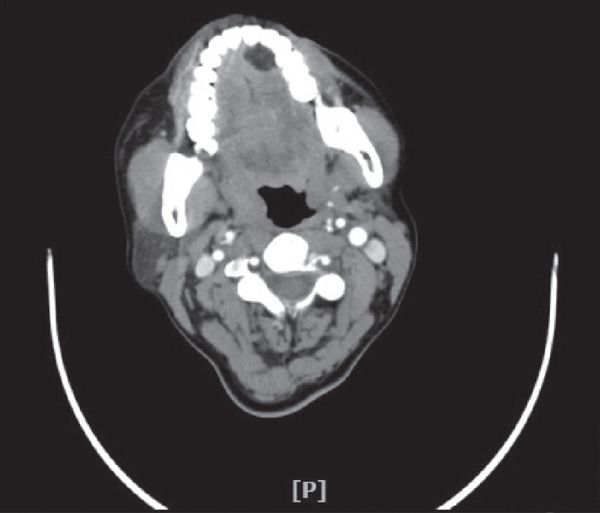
Contrast‐enhanced computed tomography (CT). A well‐circumscribed, oval, and low‐density lesion, with a few internal septations, approximately 1.5 cm in diameter, is appreciable on the left lateral border of the tongue.
